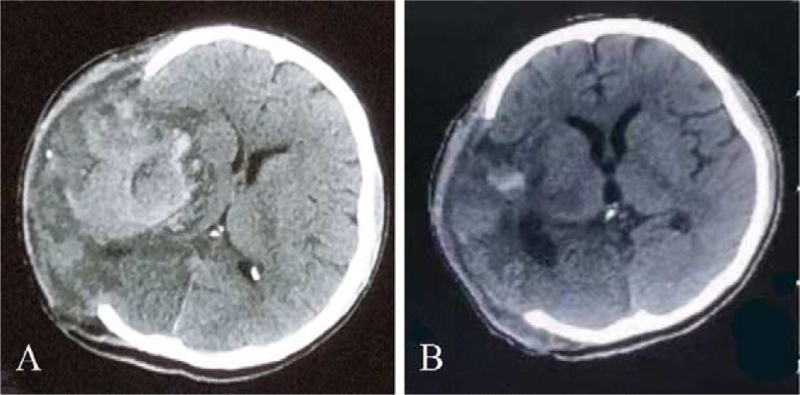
Patient's imaging data during the fourth ICH. (A) The head CT showed ICH in the right basal ganglia region again. (B) Postoperative head CT showed that hematoma was well cleared. CT = computer tomography, ICH = intracerebral hemorrhage.
